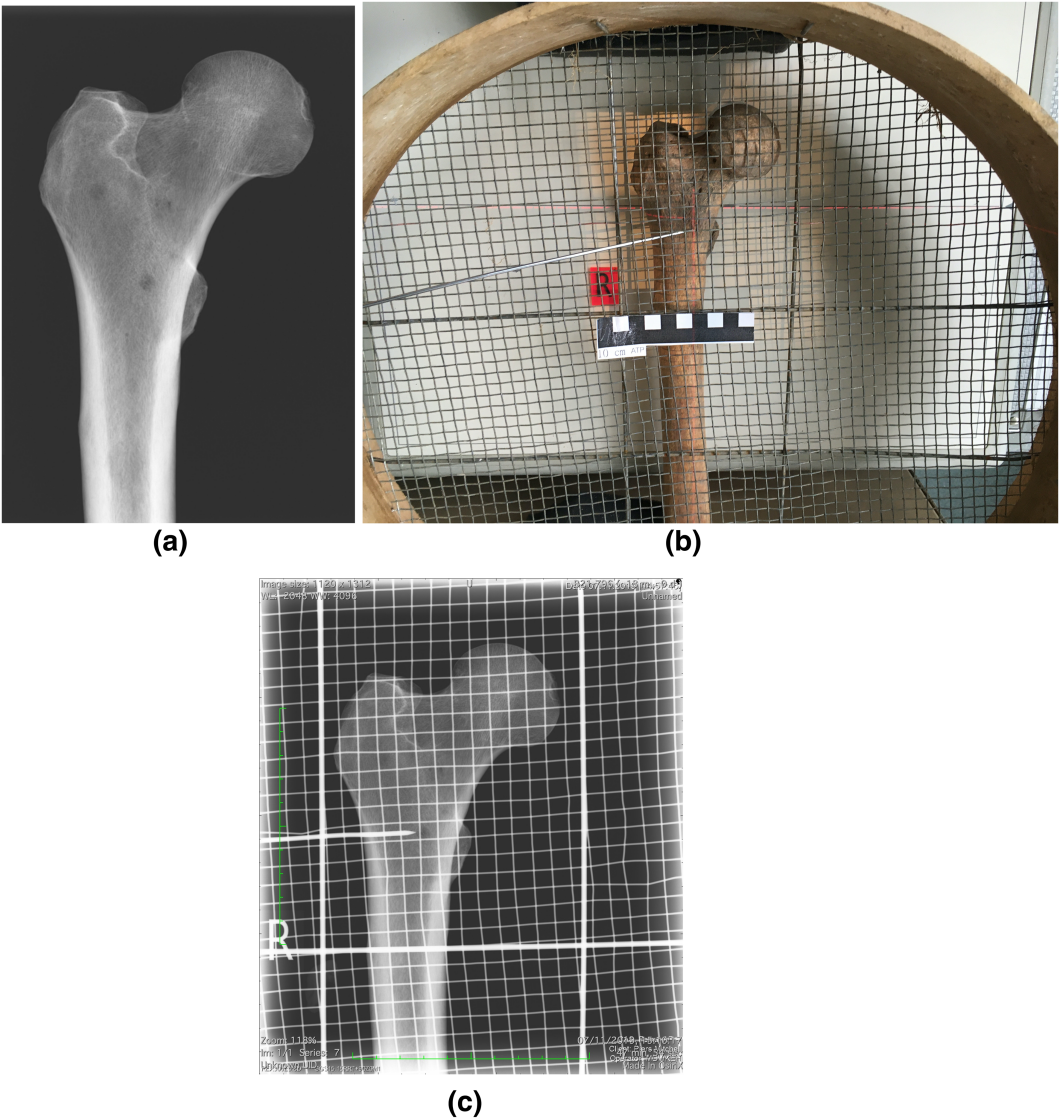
(a) Plain radiograph of right proximal femur of PSN76 showing multiple lytic metastases. (b) Position of the sieve over the femur placed on the digital radiography plate. (c) Radiograph of the femur with pointer highlighting the metastasis prior to marking the bone surface [Colour figure can be viewed at wileyonlinelibrary.com]
